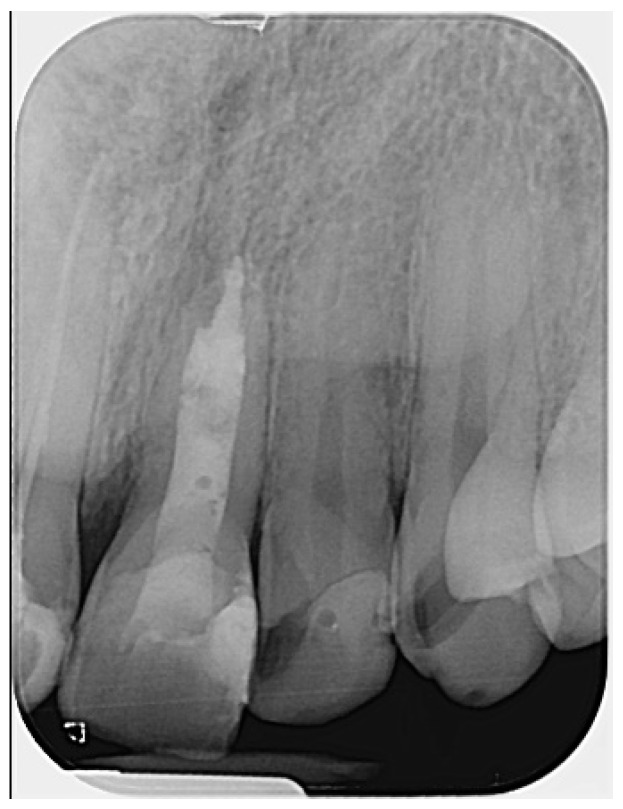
Radiograph following root canal obturation with CSBHC. Evidence of bone healing around the apex is visible, likely initiated during the period of calcium hydroxide intracanal dressing. (The mark in the lower left corner indicates the left side).
